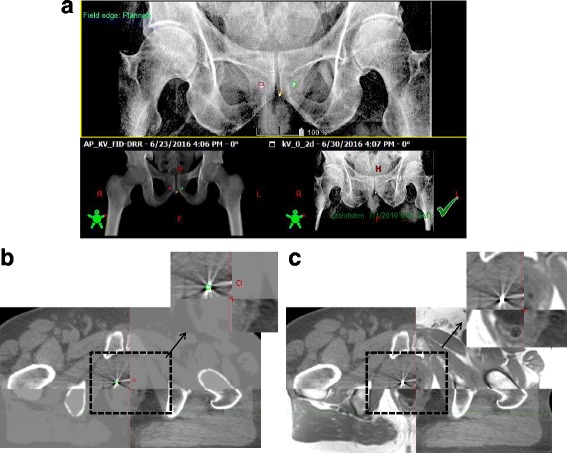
Treatment localization in Offline Review performed using: a 2-D kV DRRs from OBI and MRCAT syn-CT (b) 3-D match between CBCT and MRCAT syn-CT, (c) 3-D match between CBCT and MRCAT source MR using the MRCAT in-phase MR as a reference image
